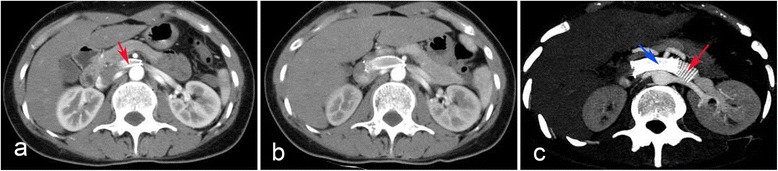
The images of the computed tomographic angiography (CTA). a, The second CTA evaluation was suggestive of an endovascular stent migration (red arrow) on the left of the superior mesenteric artery. b, The third CTA demonstrated further migration of the endovascular stent on the left of SMA. c, The follow-up CTA demonstrated the extravascular stent (red arrow) was patent and well positioned, and the endovascular stent (blue arrow) remained on the left of the superior mesenteric artery
